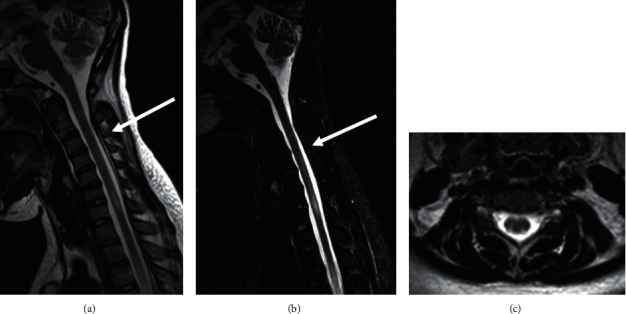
Follow-up MRI of the cervical and thoracic spine two months after treatment. (a) Sagittal STIR and (b) T2-weighted MRI images show interval improvement of the previously seen abnormal spinal cord signal intensity. (c) Axial T2-weighted image at C4 level demonstrates interval improvement of the previously seen abnormal signal intensity.
